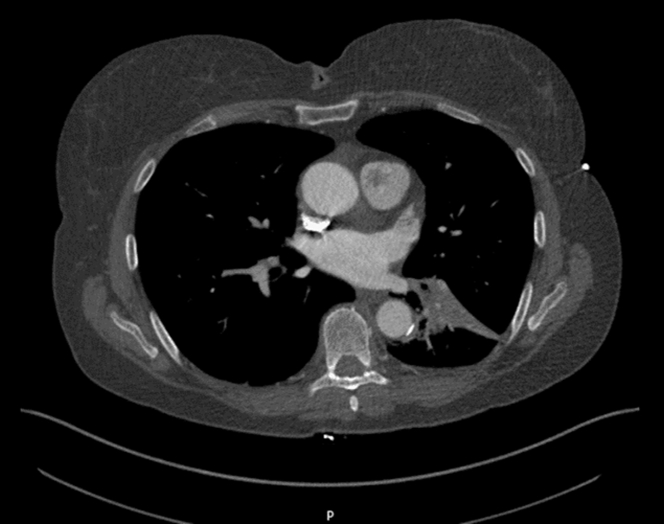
Computed Tomography Pulmonary Angiogram Showing Filling Defect in the Pulmonary Artery
